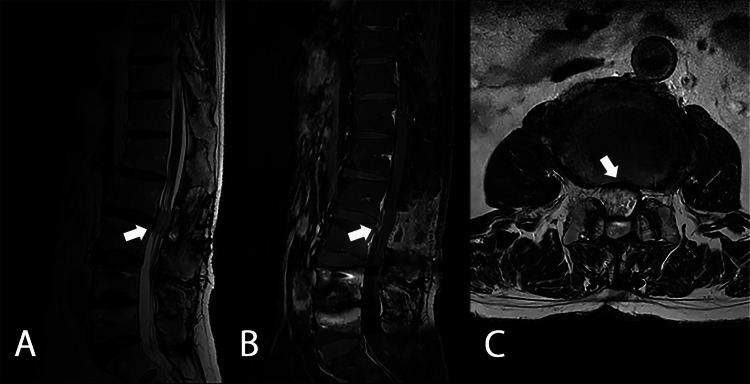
MRI scans three months after the removal of L2/3 mass lesion and immunosuppressive therapy A: T2WI sagittal section; B: T1WI with contrast enhancement sagittal section; C: T2WI axial section. The hypertrophic dural lesion almost disappeared (white arrows) MRI: magnetic resonance imaging
